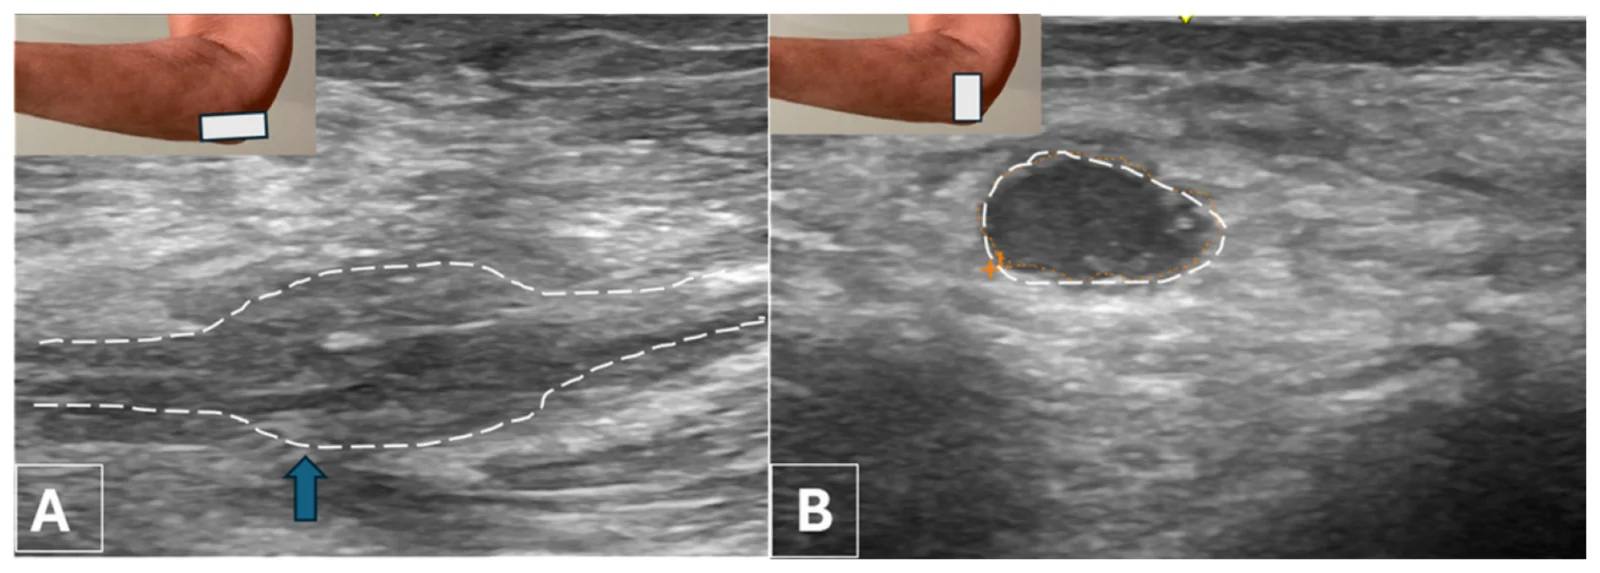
Case #2: Stab injury at the medial side of the elbow resulting in acute ulnar neuropathy. Ultrasound study: (A) Long-axis view showing a neuroma in continuity with questionable fascicular continuity (arrow). (B) Short-axis view of the ulnar nerve at the medial epicondyle showing an increase in cross-sectional area. The ulnar nerve is hypoechoic.
